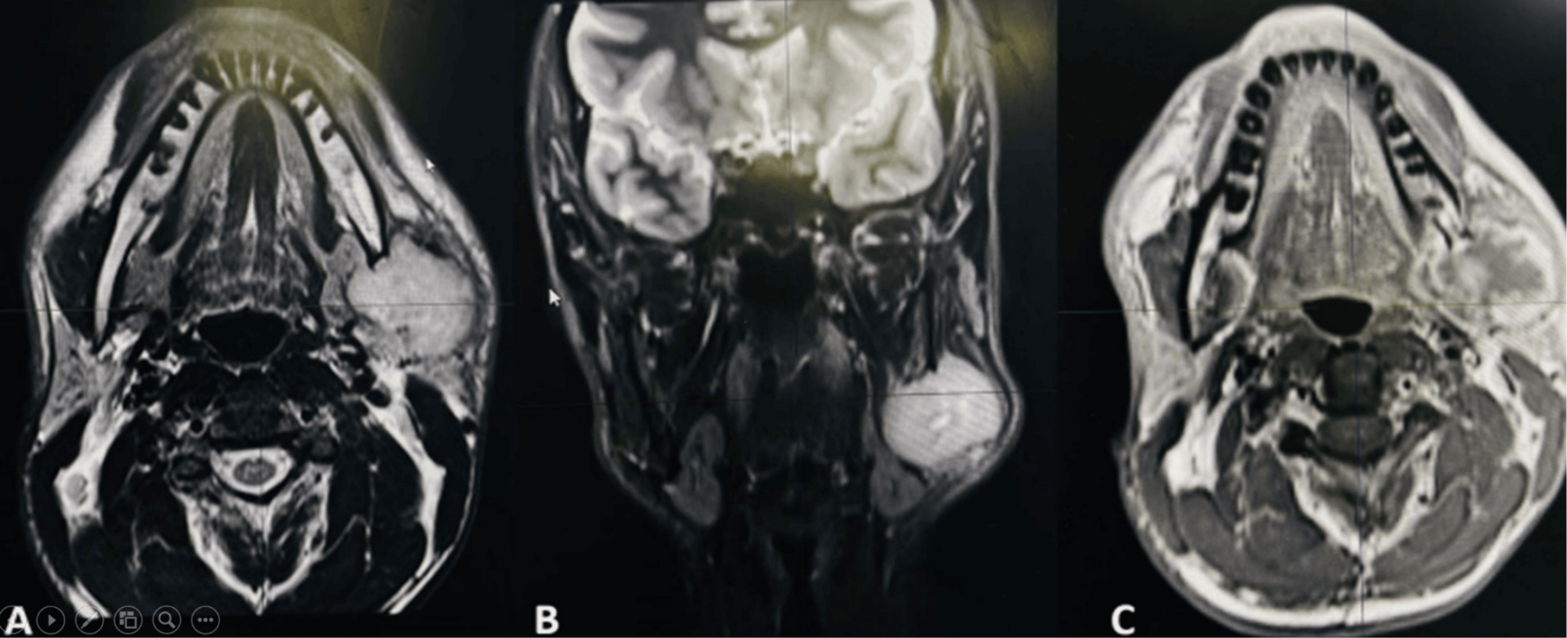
(A, B) T2 axial and coronal MRI revealed a 3.7 x 3.8 cm mass lesion originating from the angle of the mandible on the left side, associated with a soft tissue component involving both sides of the bone. The mass was observed to invade the left masseter muscle and left medial pterygoid muscle, (C) T1 axial MRI revealed mass originate from the angle of mandible and invaded the adjacent muscle medially and laterally.
